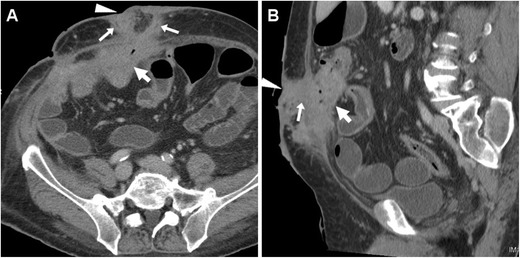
Ileal adenocarcinoma fistulising to the skin in a 68-year-old man with long-standing CD, previous ileocecal resection, recent weight loss and two draining EFOs at the anterior abdominal wall. CT-enterography confirmed double ventral ECF (arrows) from a markedly thickened non-stratified ileal segment (thick arrows). Neoplastic infiltration of the anterior abdominal wall was pathologically confirmed after en-bloc surgical resection. Tumour progression with ascites and liver metastases (not shown) ultimately developed (Partially reproduced from Open Access ref. no [34])
